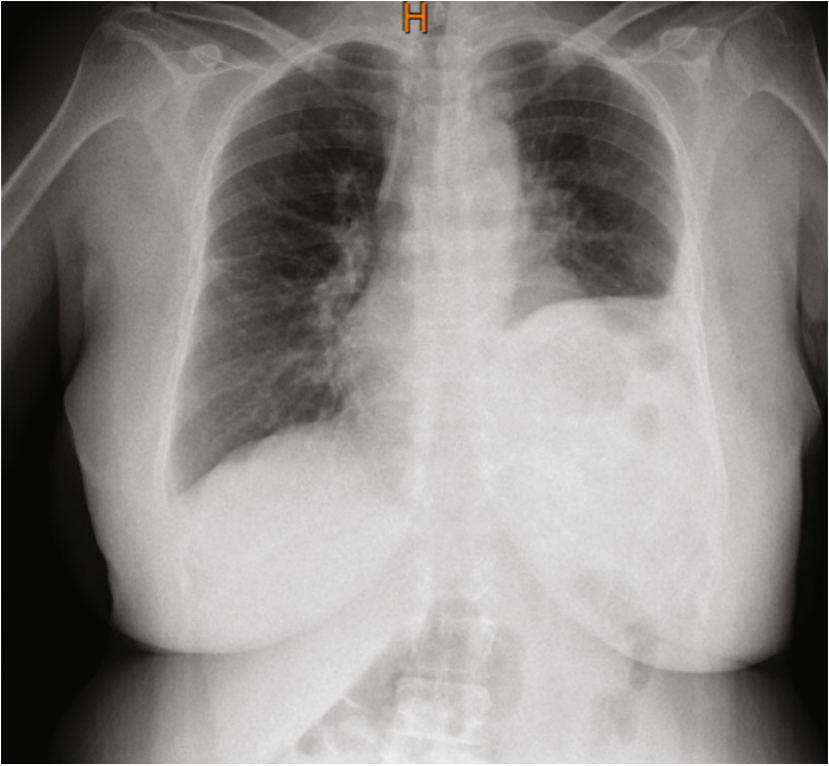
Chest X-ray of our patient on the day that she presented with dyspnea and chest pain.
